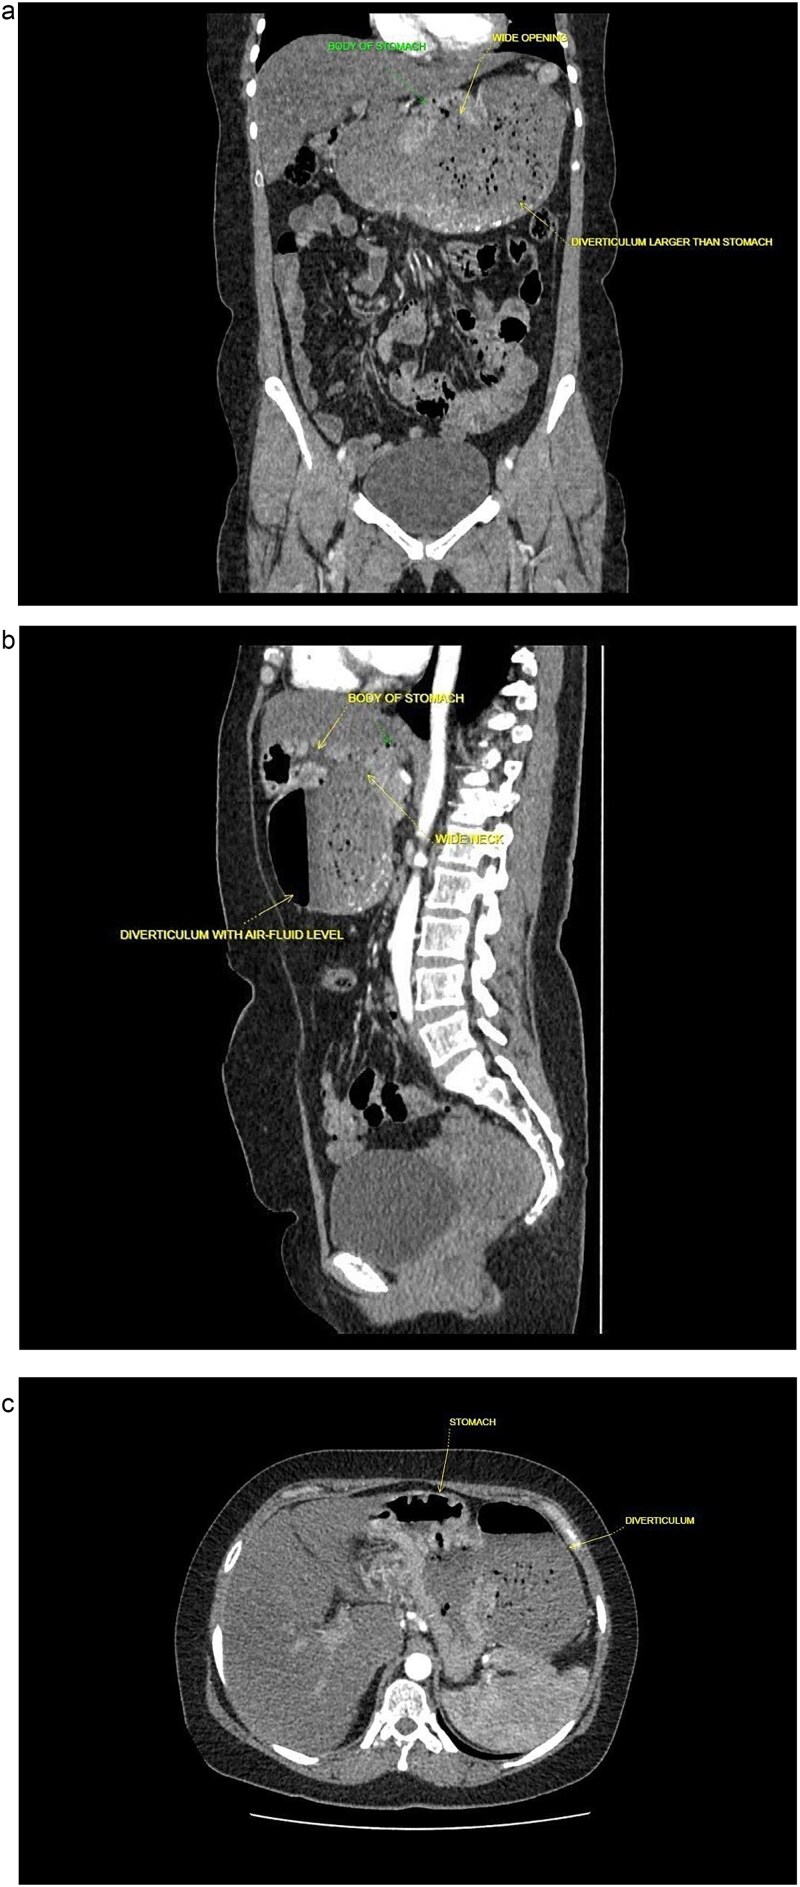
Contrast enhanced computed tomography (CECT) of abdomen and pelvis. (a) Sagittal plane. (b) Coronal plane. (c) Axial plane.
